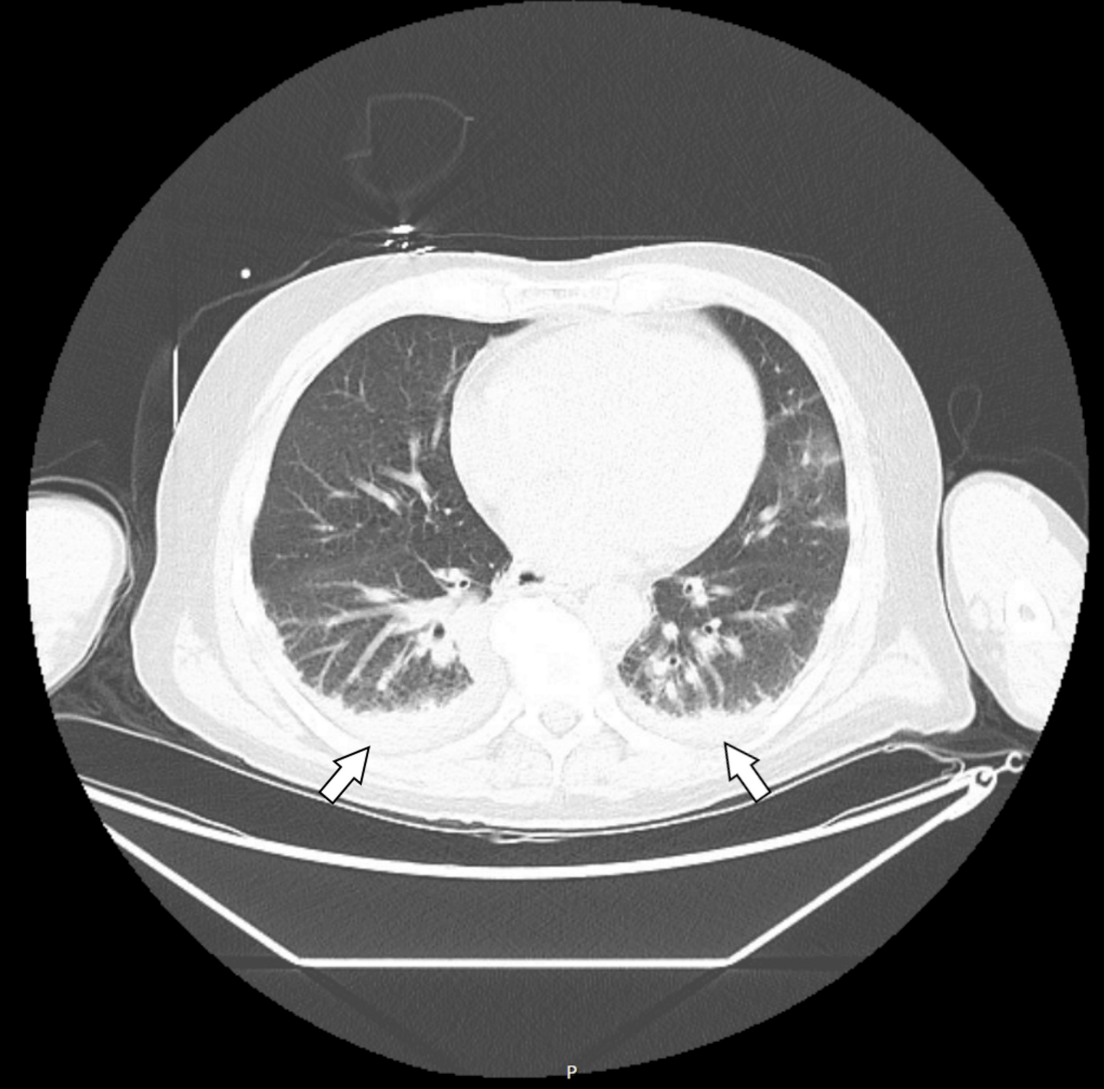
CT scan of the chest at admission showing bilateral lower lobe atelectasis and pleural effusions The arrows point to the pleural effusions. CT: Computed tomography.
